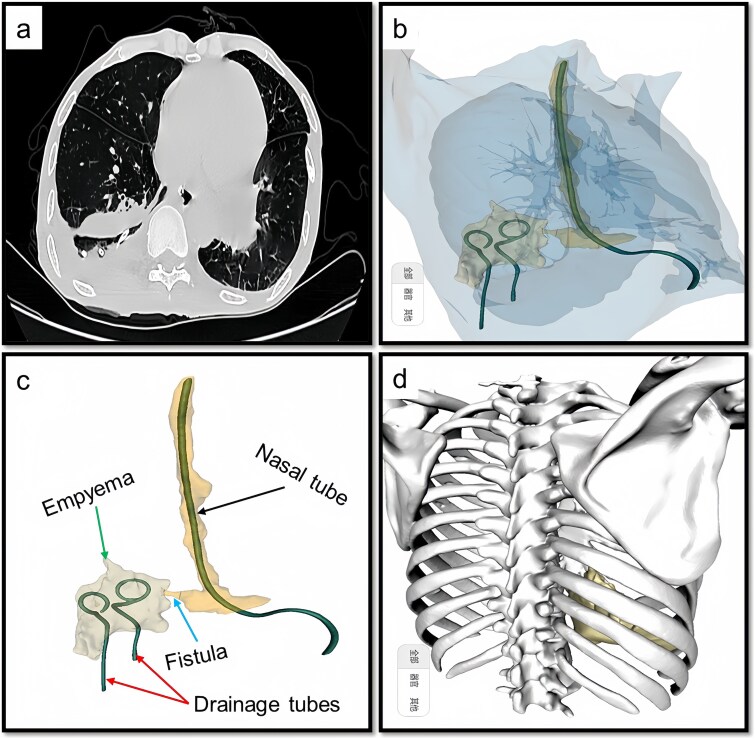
Preoperative CT and 3D reconstruction images. (a) CT revealed that the fistula and associated cavity remained unchanged. (b) 3D reconstruction provides a comprehensive visualization of the fistula and its associated cavity. (c) Demonstration of the details, we can see the fistula (arrow), empyema, nasal tube, and drainage tubes. (d), 3D reconstruction including bones, the 10th rib is situated at the center of the abscess, and accordingly, the extent and number of rib dissections are determined based on this anatomical reference.
